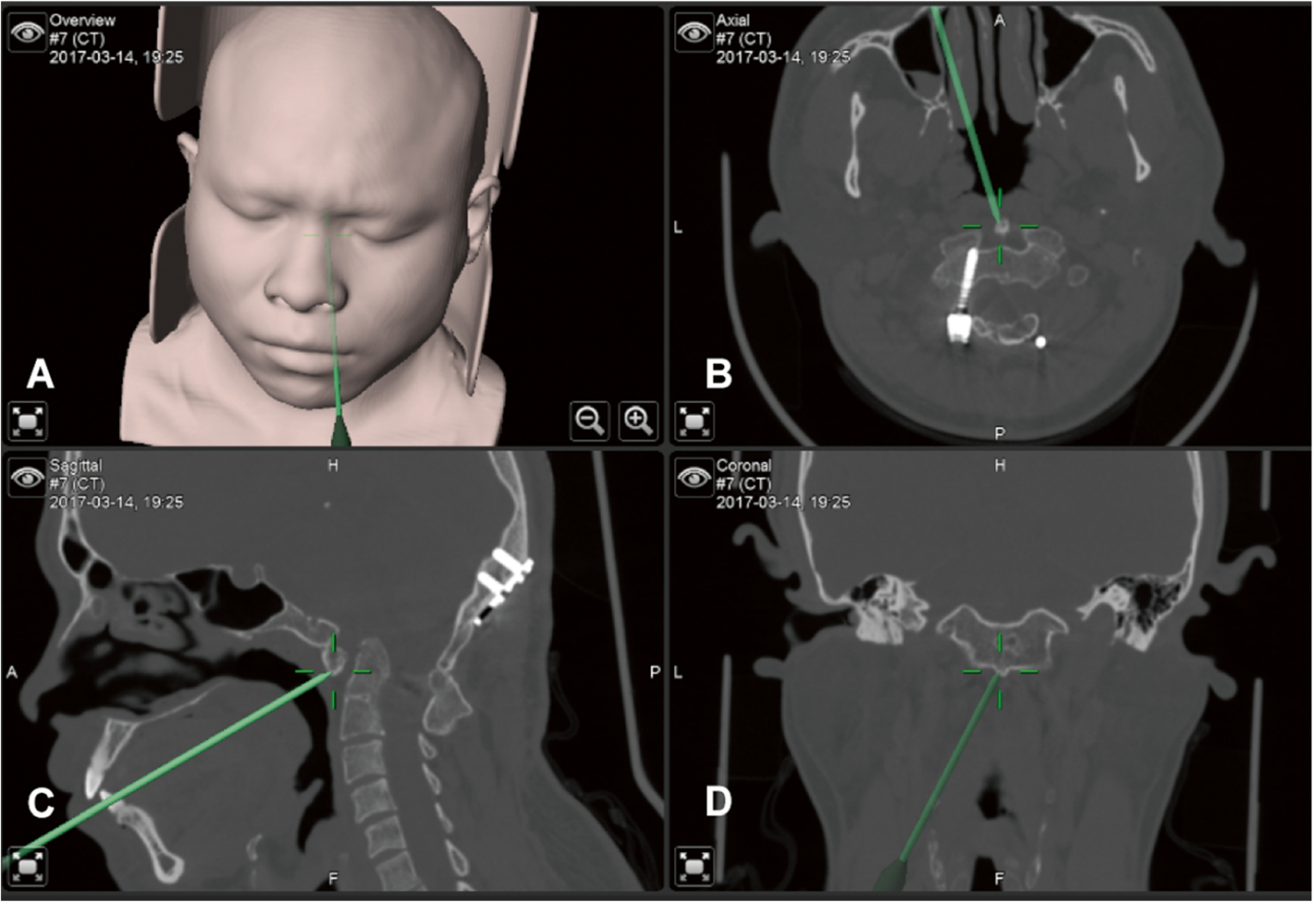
The incision on the posterior pharyngeal wall, anatomical landmarks, and the resection range of the odontoid process were determined according to the navigation. a Facial surface registration. b, c, d Axial, sagittal, coronal CT navigation image indicating the midpoint of anterior C1 arch lower margin
